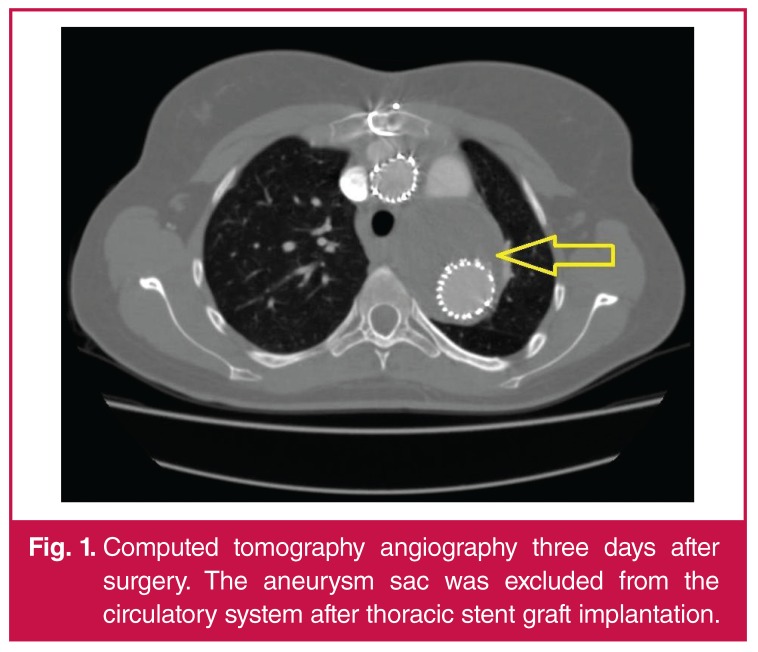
Computed tomography angiography three days after surgery. The aneurysm sac was excluded from the circulatory system after thoracic stent graft implantation.
